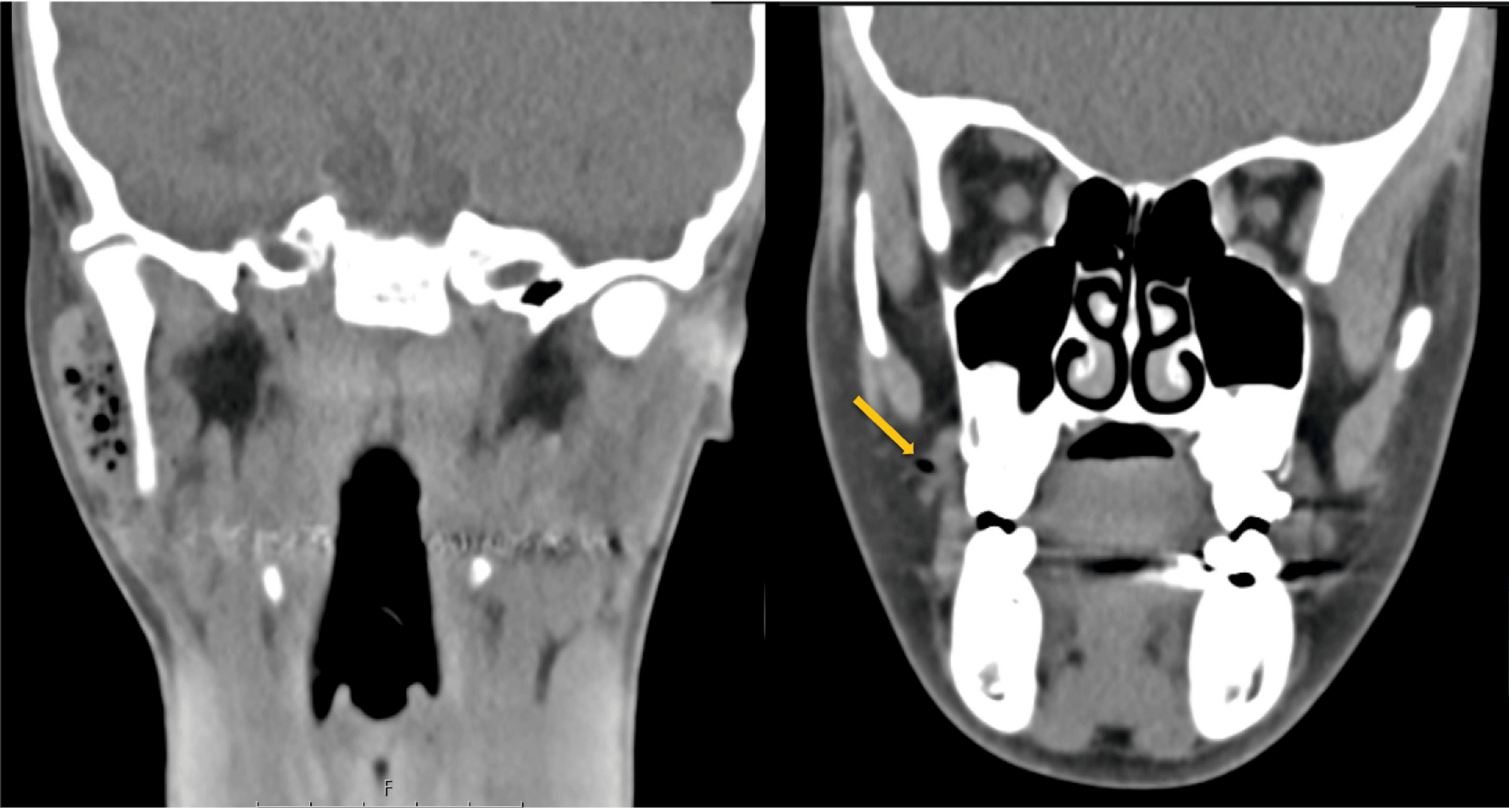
CT scan Coronal CT sections demonstrate enlargement of the right parotid gland with multiple millimeter-sized intraglandular gas bubbles and no signs of inflammation. Gas is also observed within Stensen’s duct, extending to the level of the buccinator muscle (arrow).
